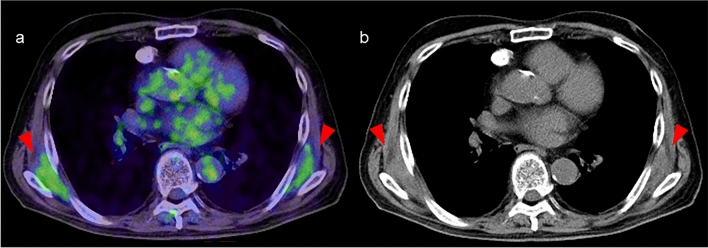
a Axial image of FDG-PET/CT and b CT image. Elastofibroma dorsi is typically characterized by mild to moderate FDG uptake, seen here in a soft tissue mass located around the bilateral inferior scapular angles (arrowhead)
